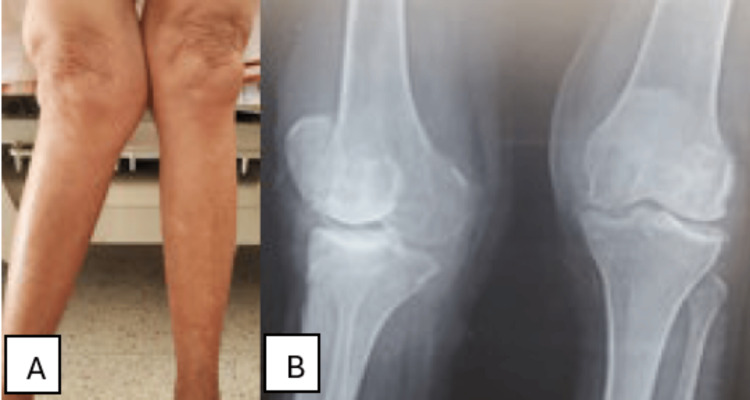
Imaging of Patient 2. (A) Clinical photograph of the knees demonstrating knee deformity. (B) Anteroposterior radiograph of both knees revealing bilateral destruction and malalignment.
